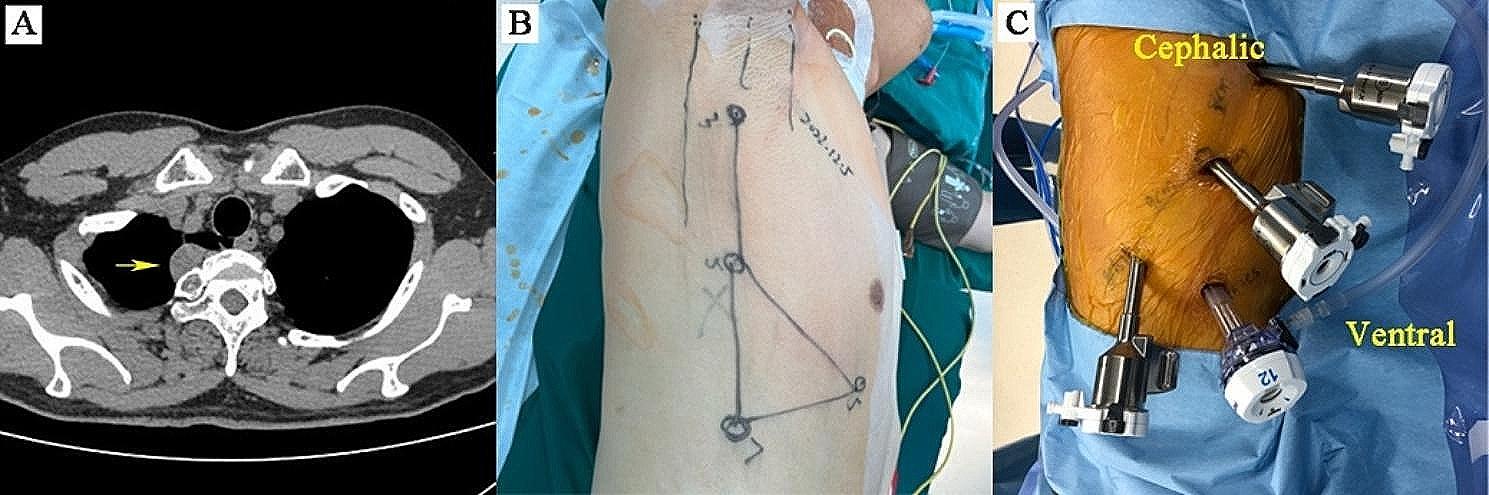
Chest CT image, operating position, and port location for robotic posterior mediastinal tumour surgery. (A) The preoperative computed tomographic scan revealed a suspected schwannoma on the right thoracic paravertebral side. (B, C) Lateral decubitus position
